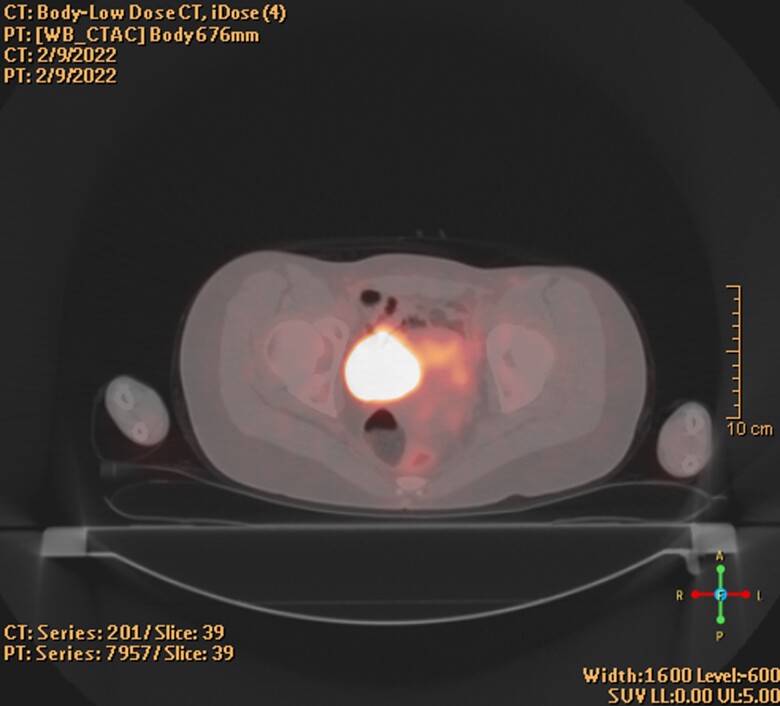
Positron emission tomography-computed tomography scan with DOTATATE staging. Increased tracer uptake in the right superolateral region of the urinary bladder.
